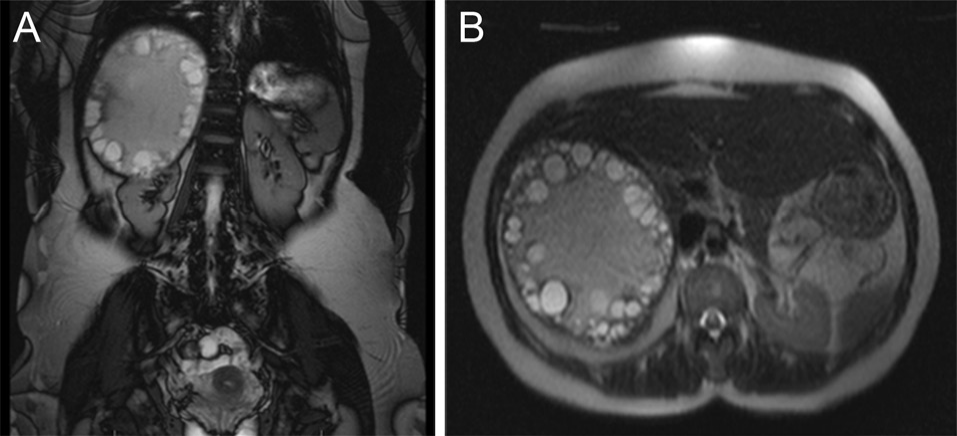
Coronal (A) and axial (B) T2-weighted magnetic resonance image of the abdomen demonstrating right adrenal cystic mass with daughter cysts inside a mucinous or solid cyst matrix.
